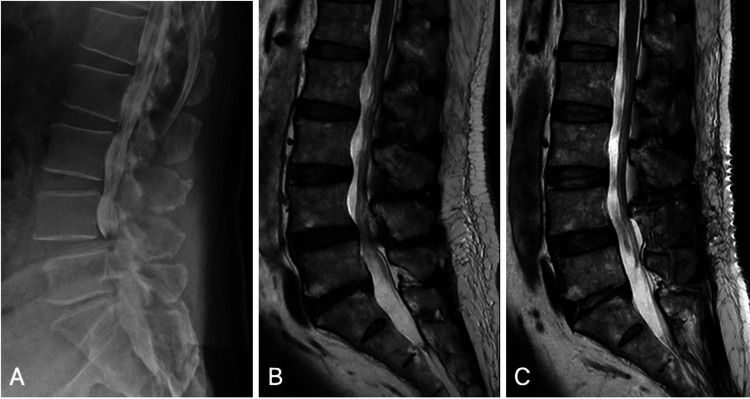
Preoperative myelography and changes in T2-weighted images before and after surgery. A: Myelography showing a total stop of the contrast agent at L4/5. B: MRI T2-weighted images showing central canal stenosis at the L4/5 level. C: Postoperative MRI showing resolution of the previous lumbar canal stenosis.
